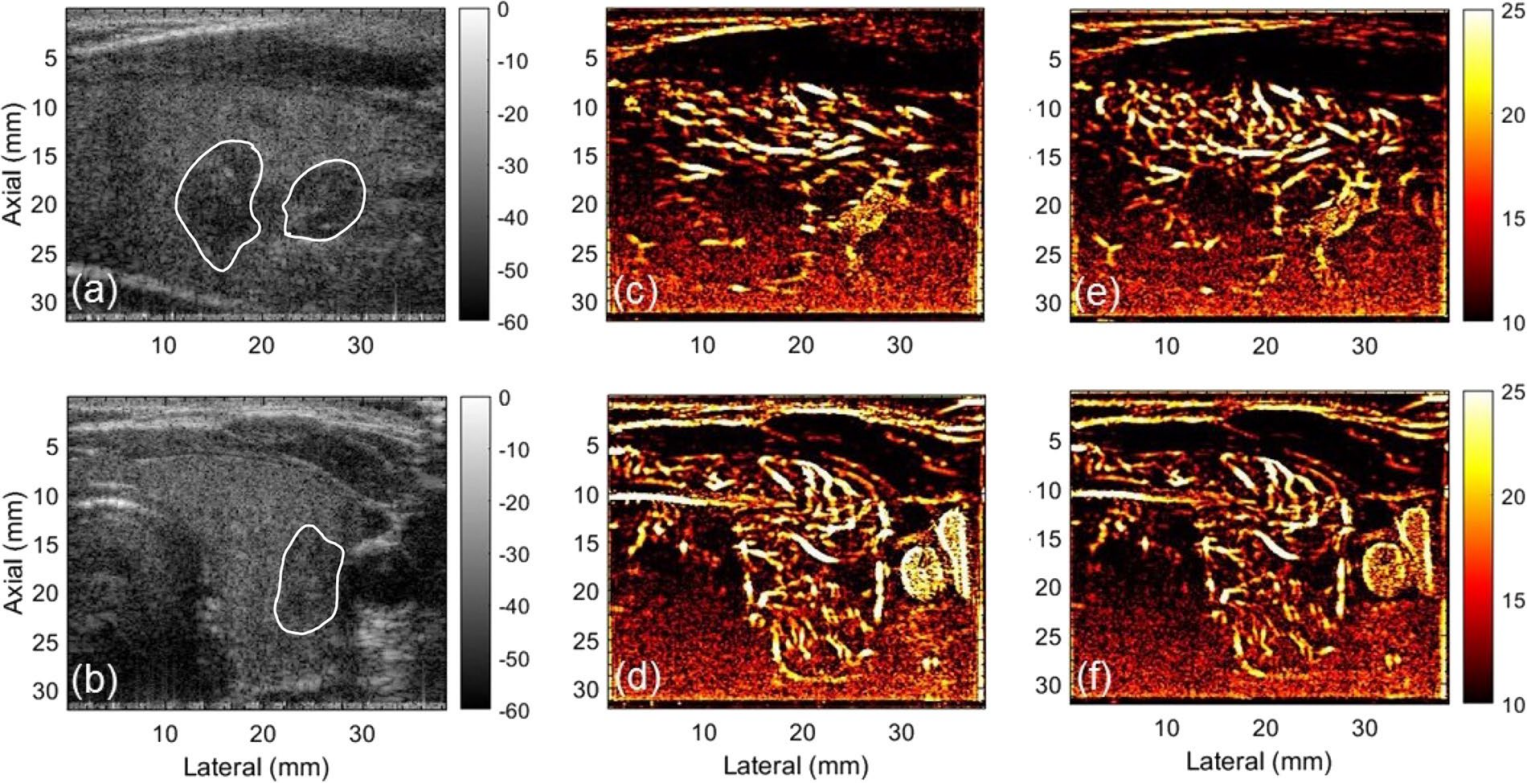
Displays the plane wave B-mode sonograms (a,b) and the corresponding PD images – without (c,d) and with (e,f) motion correction. (a,c,e) corresponds to the longitudinal plane, and (b,d,f) corresponds to transverse plane. For the sake of clarity in visualizing the small blood vessel structures, the tumor outline is indicated on the B-mode sonogram.
